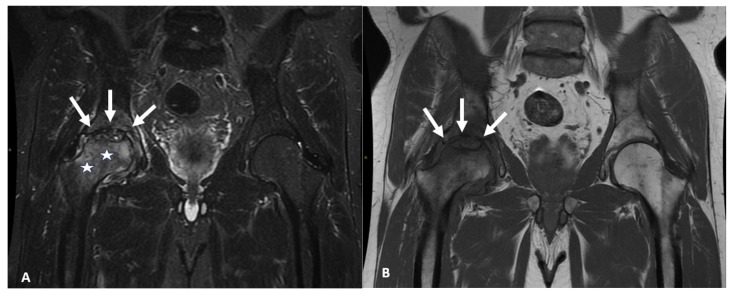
(A): STIR coronal view of a 50-year-old male, after severe COVID-19, 42 days in ICU and 1315 mg of methylprednisolone in 45 days. At the time of MRI, the patient was symptomatic (right groin pain after a few meters walk). Typical appearance of femoral head ON on the right side (arrows), associated with locoregional bone marrow edema of the neck-trochanteric region (stars). (B). T1 coronal view of the same patient of Figure 1, which shows partial collapse of the right femoral head (arrows).
